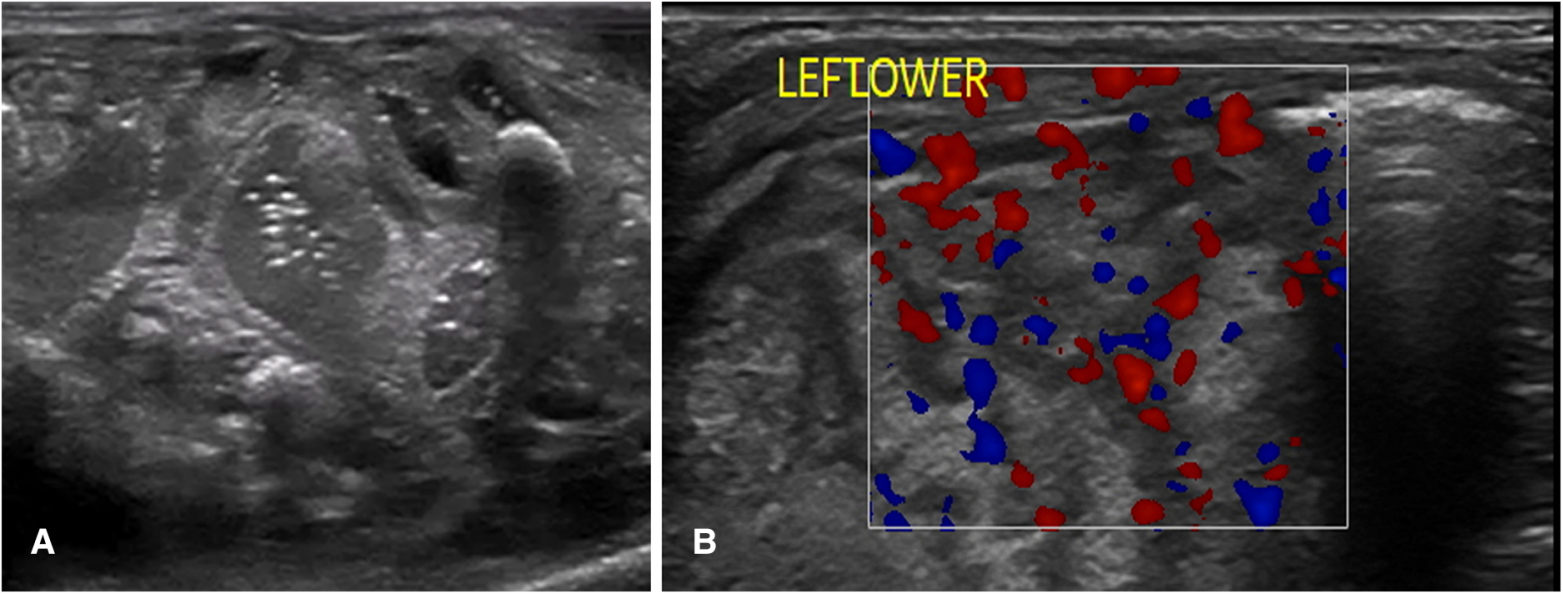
The sonographic appearance of normal bowel. (A) 2-D Grayscale image of normal bowel with normal intraluminal air seen as echogenic dots. (B) Color Doppler imaging of bowel showing normal bowel vascularity.
